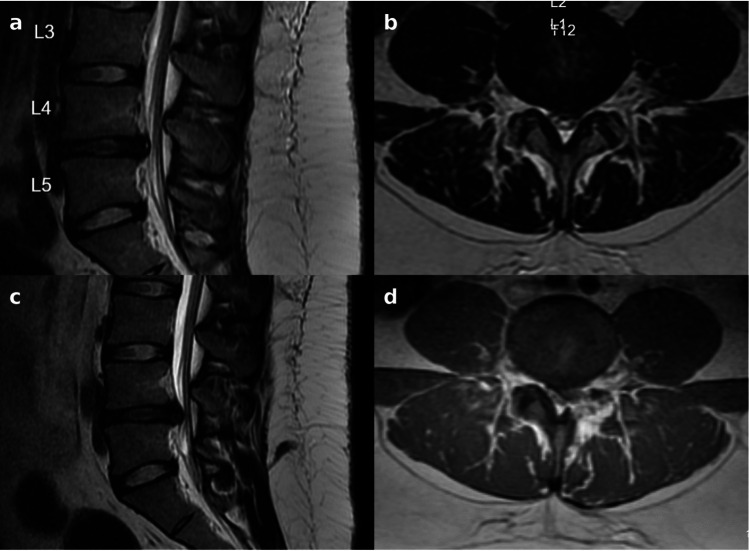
Pre- and postoperative MRI of an 18-year-old female with a left L4–5 lumbar disc herniation and CSS treated by left L4–5 minimally invasive microdiscectomy (MIS-MCD), with recurrent left L4–5 herniation three years after the index procedure. (a) Preoperative T2-weighted sagittal MRI; (b) preoperative T2-weighted axial MRI; (c) T2-weighted sagittal MRI at recurrence; (d) T2-weighted axial MRI at recurrence
